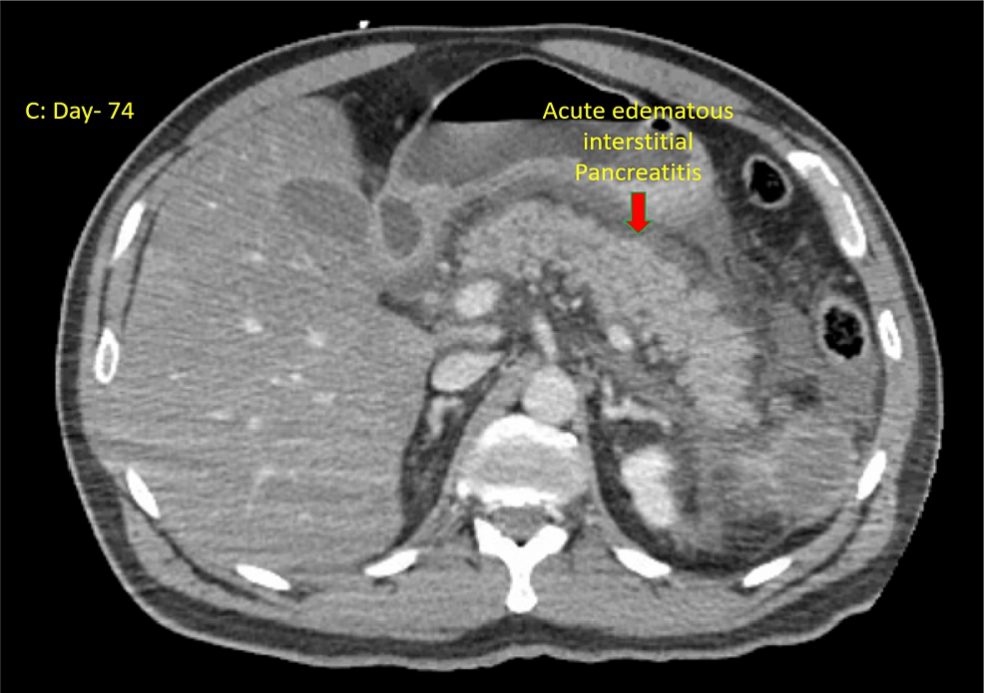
Acute edematous interstitial pancreatitis seen on a CT scan of the abdomen.
